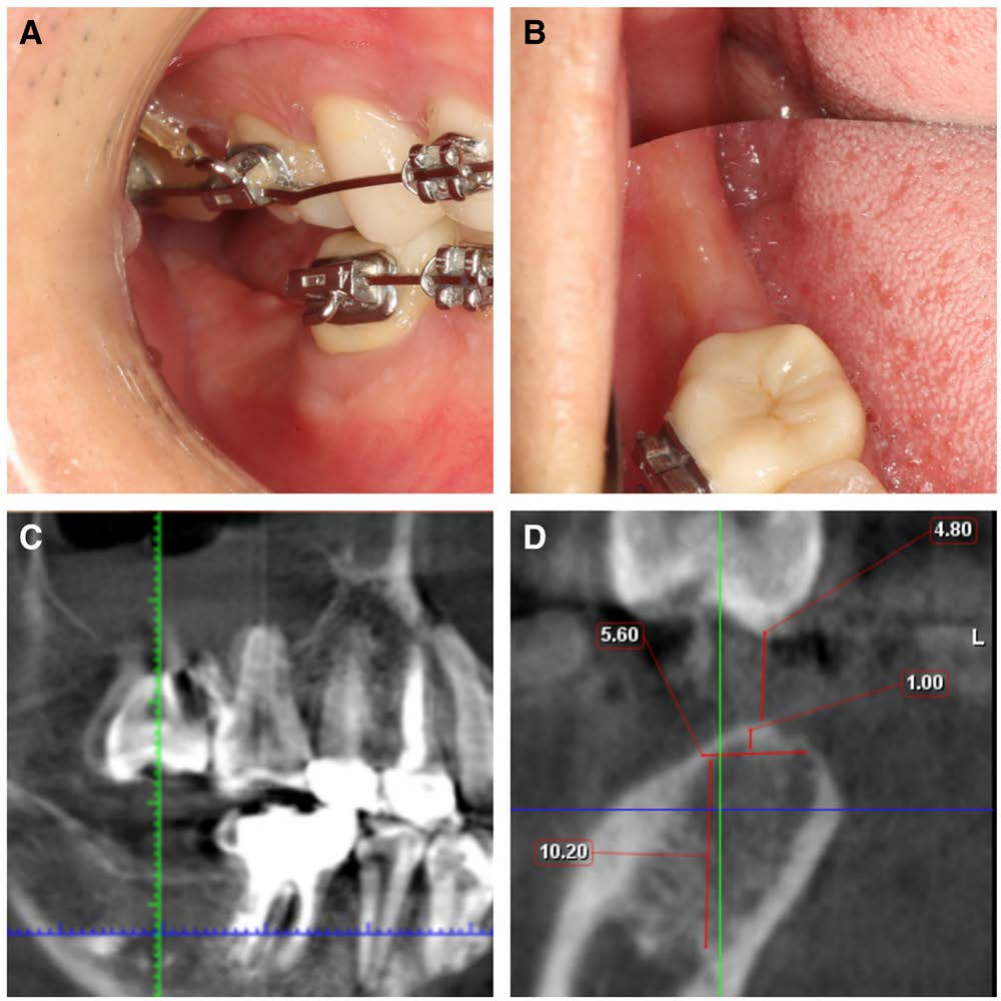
Preoperative assessment. (A) Right occlusion photo. (B) Occlusal photography. (C) Sagittal slice imaging of CBCT. (D) Coronal section screenshots. CBCT = cone-beam computed tomography.
